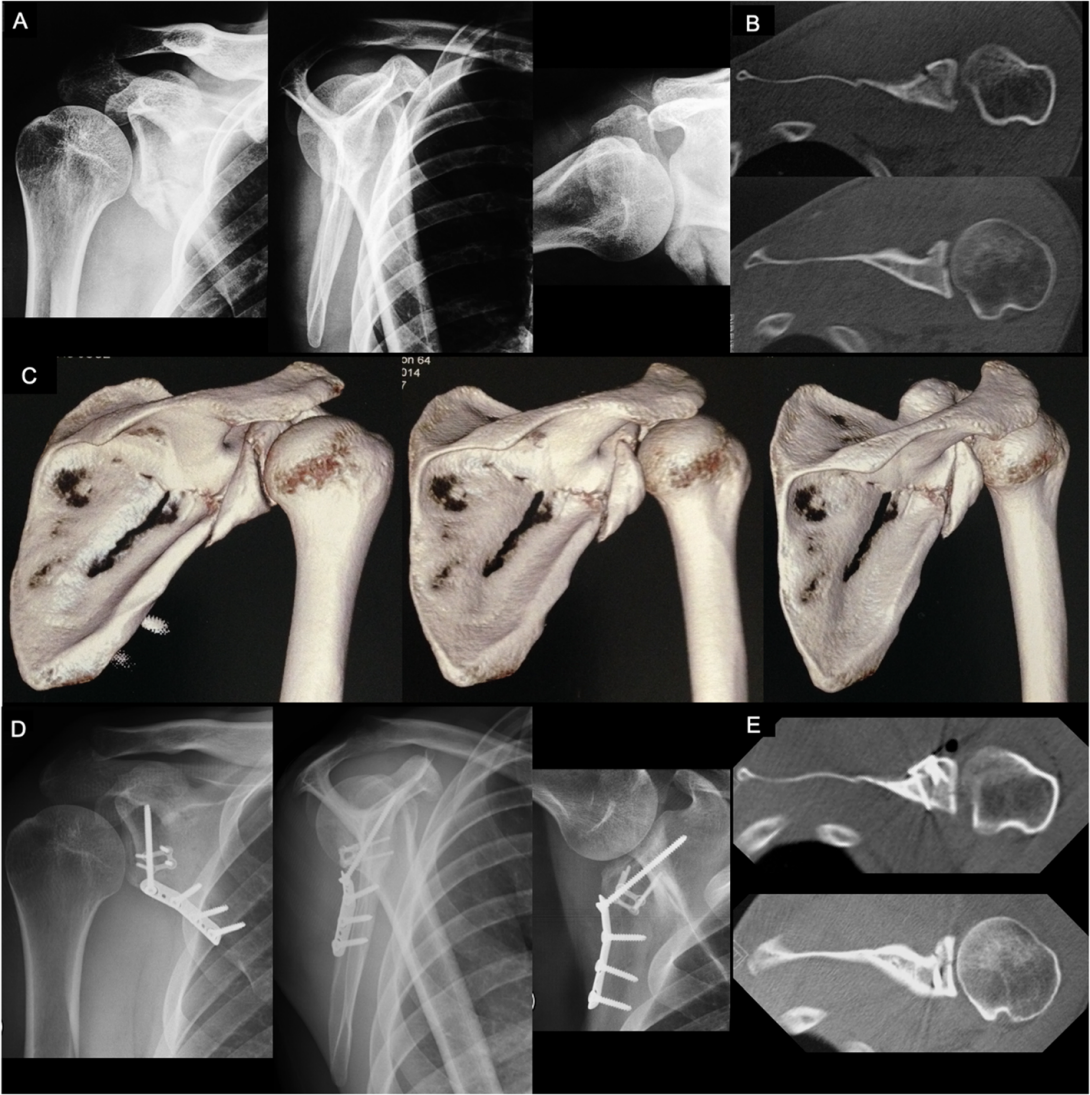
a: Preoperative true AP, lateral scapular, and axillary radiographic views of the right shoulder of a 25-years-old male patient, showing a displaced inferior glenoid fragment extending to the lateral pillar of the scapular neck and body. Patient reported on a fall from stairs 48 h before. Also note the small bone fragments in the inferior portion of the capsule (yellow arrowheads); b: Preoperative CT axial cuts of the right shoulder demonstrating the displaced inferior glenoid fracture; c: Preoperative 3-D CT reconstructions showing the displaced inferior glenoid fracture extending to the lateral pillar of the scapular neck and body; d: Postoperative true AP, lateral scapular, and axillary radiographic views of the right shoulder demonstrating the anatomic reduction of the inferior glenoid fracture and buttressing with a one-third tubular plate. Observe the 2.3-mm reduction plate used to maintain the reduction during surgery. Note the long 3.5-mm screw inserted through the plate directed to the coracoid process; e: Postoperative CT axial cuts of the right shoulder demonstrating the anatomic reduction of the inferior glenoid fracture
